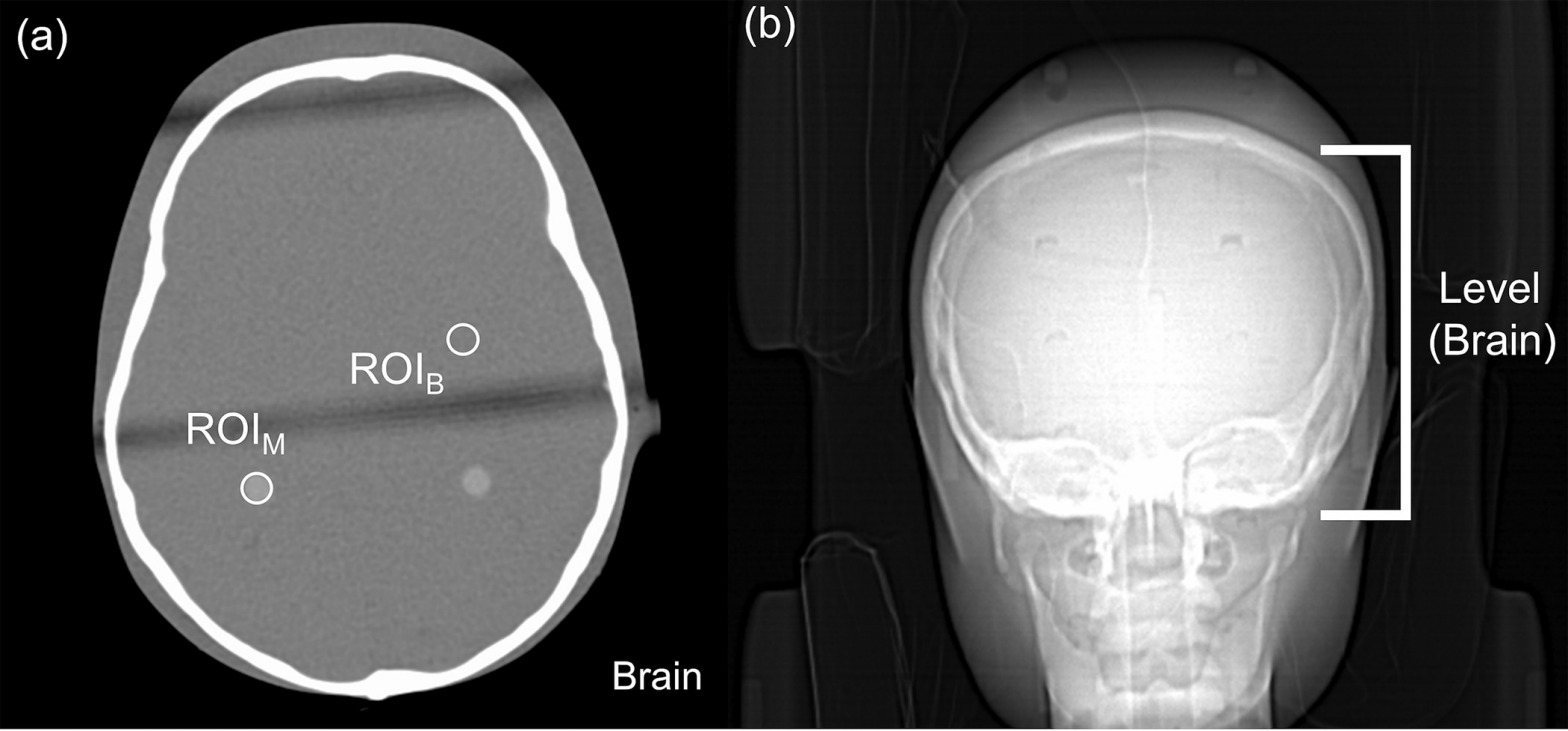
Determination of the axial image quality for the 1-year-old AP brain (window width/level = 400/40) (a) Two 20-mm^2^ areas of brain parenchyma were encircled as regions of interest (ROIs), and the mean CT number (CT#_M_) and its standard deviation (SD_B_) (Hounsfield Unit, HU) were determined for the ROIs. The standard deviation (SD, HU) of the mean CT number (CT#_M_) within the ROI over the target region (ROI_M_) and background (ROI_B_) (b) The brain level.
